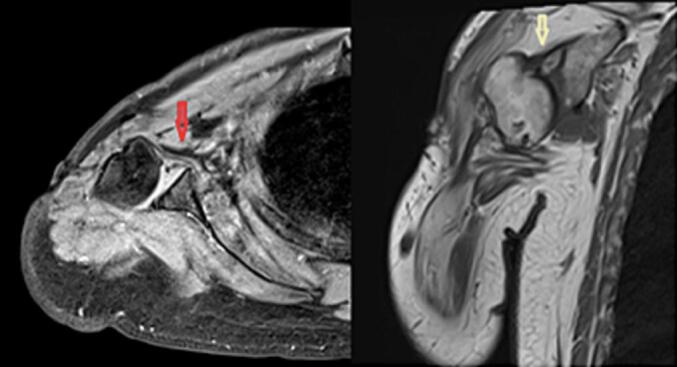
MRI image of the patient's shoulder joint, subscapularis tendon (red arrow), supraspinatus tendon (gray white arrow).
